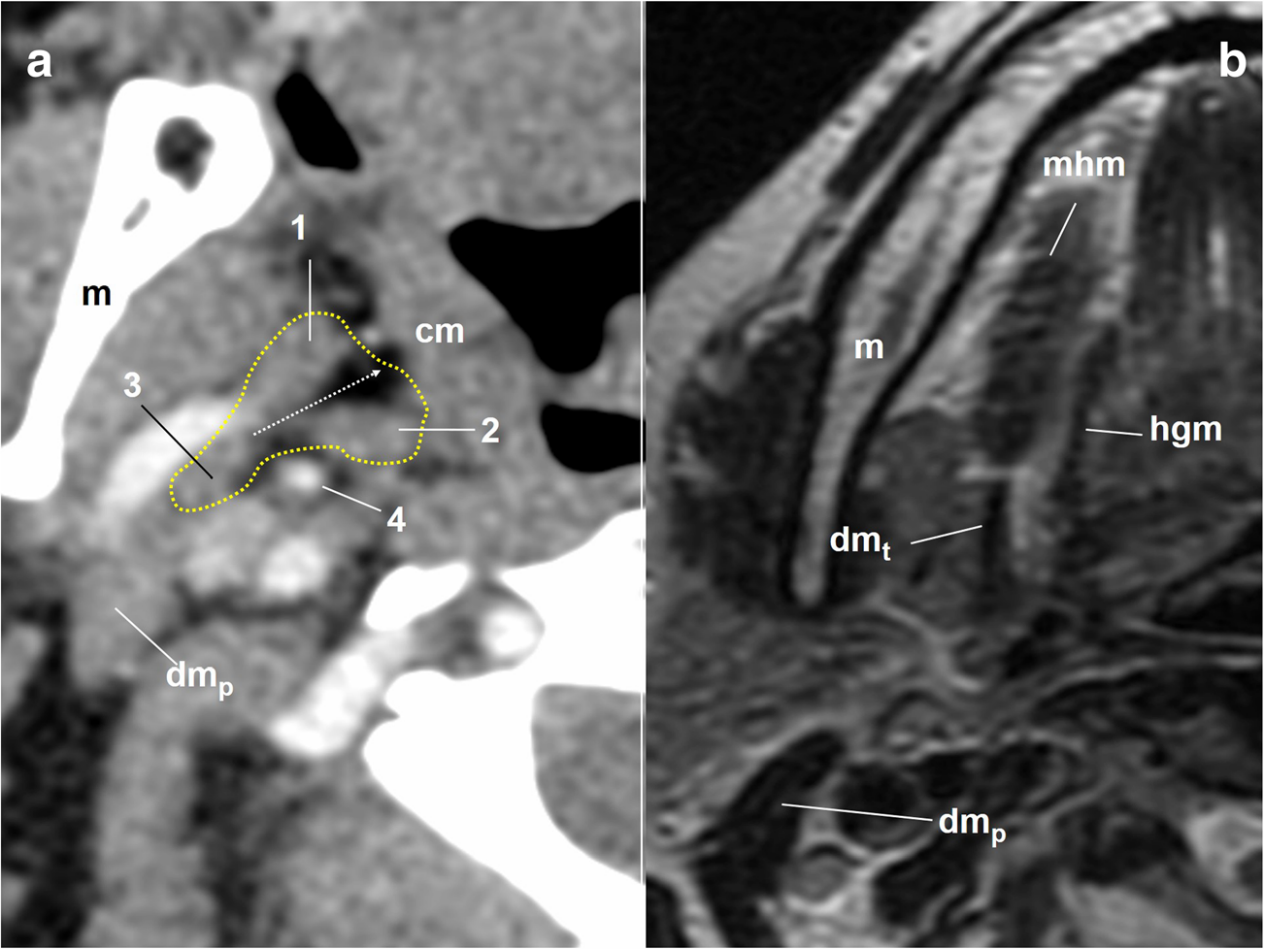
Cervical portion of the glossopharyngeal nerve. a Axial contrast-enhanced CT scan of the neck, right side, soft tissues window. The dotted yellow line draws the limits of the styloid pyramid. 1: styloglossus muscle; 2: stylopharyngeus muscle; 3: stylohyoid muscle; 4: external carotid artery; cm: constrictor muscles. The dotted white arrow represents the long axis of the styloid pyramid as a reference of the gpn pathway. dm_p_: posterior belly of the digastric muscle; m: mandible. b Axial T2-weighted MRI of the neck, right side, at the level of the intermediate tendon of the digastric muscle (dm_t_). The slice over the level of the tendon is a good reference for the entry point of the glossopharyngeal nerve in the pharynx. mhm: mylohyoid muscle
